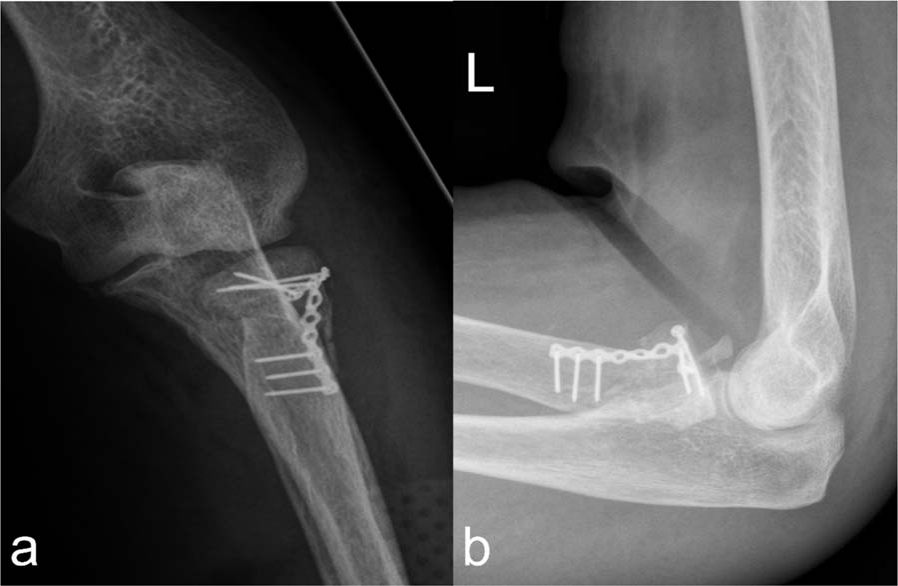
Treatment patterns of fractures of the caput and collum radii (2R1C3 and Mason stages II and III). (a, b) X-ray in two planes shows extensive coverage of the fracture fragments of the caput radii when the collum radii is straightened by the osteosynthesis material.
